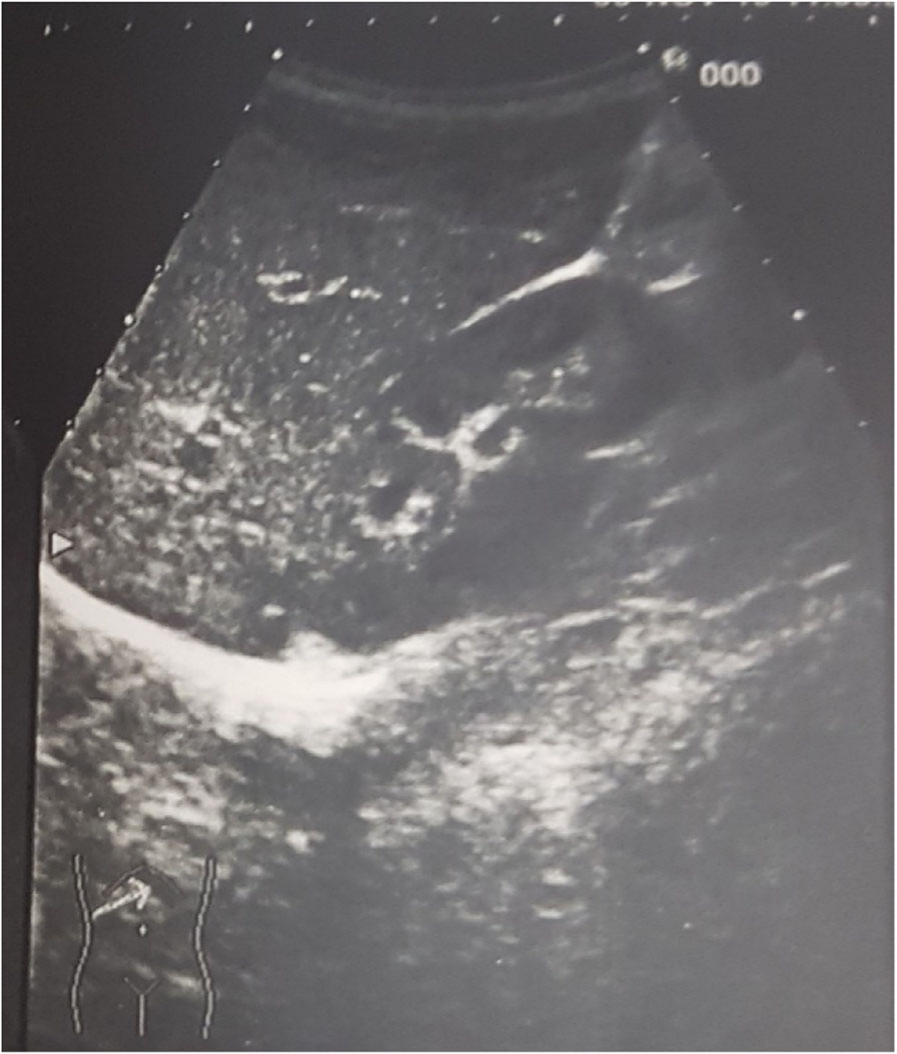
Abdomen ultrasound of the patient, showing a "starry-sky liver"
